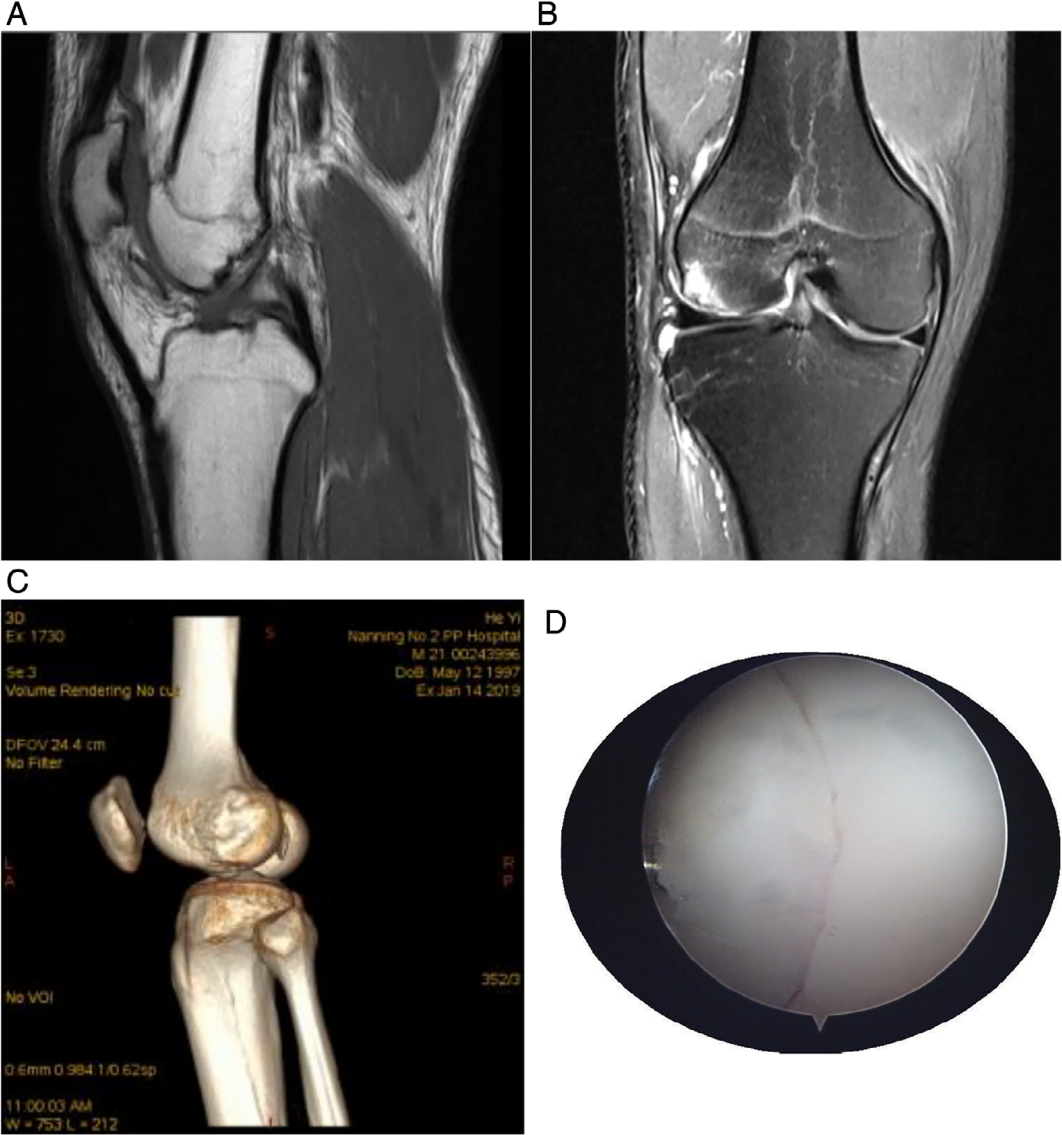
(A) MRI examination of the right knee joint: the bone continuity at the edge of the lateral condyle of the right femur was poor, the patchy high signal intensity was seen in the bone marrow cavity of the lateral condyle of the femur, and the local cartilage became thinner in the corresponding area. (B) MRI examination of the right knee joint: a patchy bone signal was seen in the anterior and lower part of the distal femur. (C) CT examination of the left knee joint: the continuity of the subarticular bone of the lateral condyle of the left femur was interrupted. (D) Under knee arthroscopy, obvious fracture line of lateral condyle of bone and osteochondral fracture of the lateral femoral condyle can be seen.
